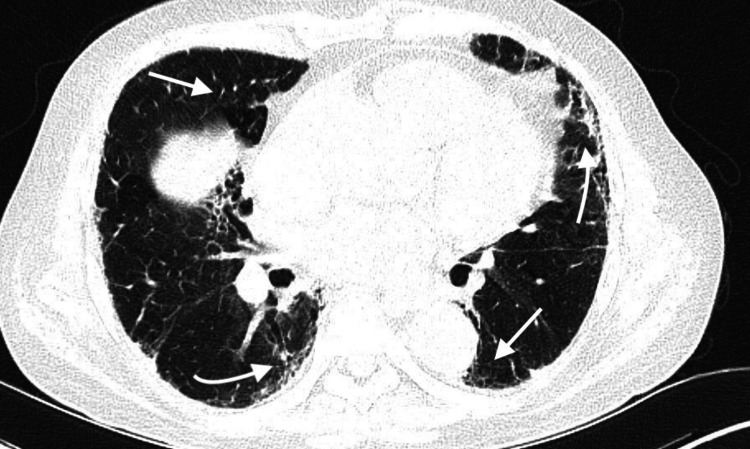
HRCT- Bilateral Inter and Intralobular septal thickening with patchy ground-glass opacities HRCT: high-resolution computed tomography
